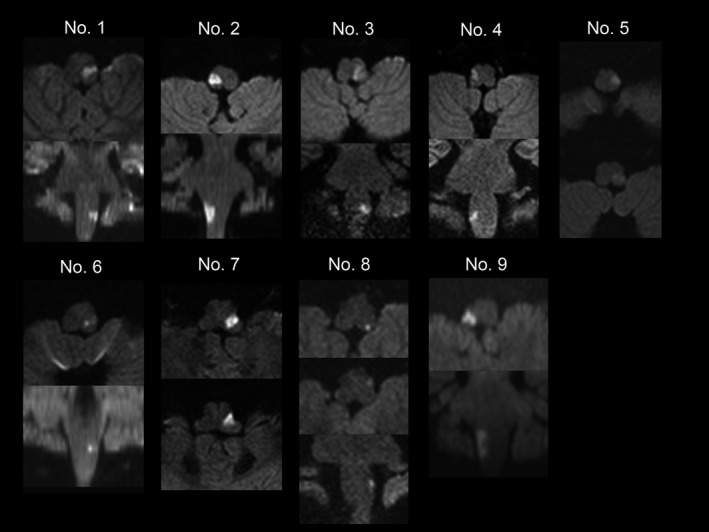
MRI findings at the medulla. The DWI high‐intensity lesion in Patients No. 1, 2, and 9, who showed a whole‐body laterality of BST, was craniocaudally longer than all other patients. In contrast, DWI high‐intensity lesion in Patient No. 6, who showed no laterality of BST, was the smallest of all patients
